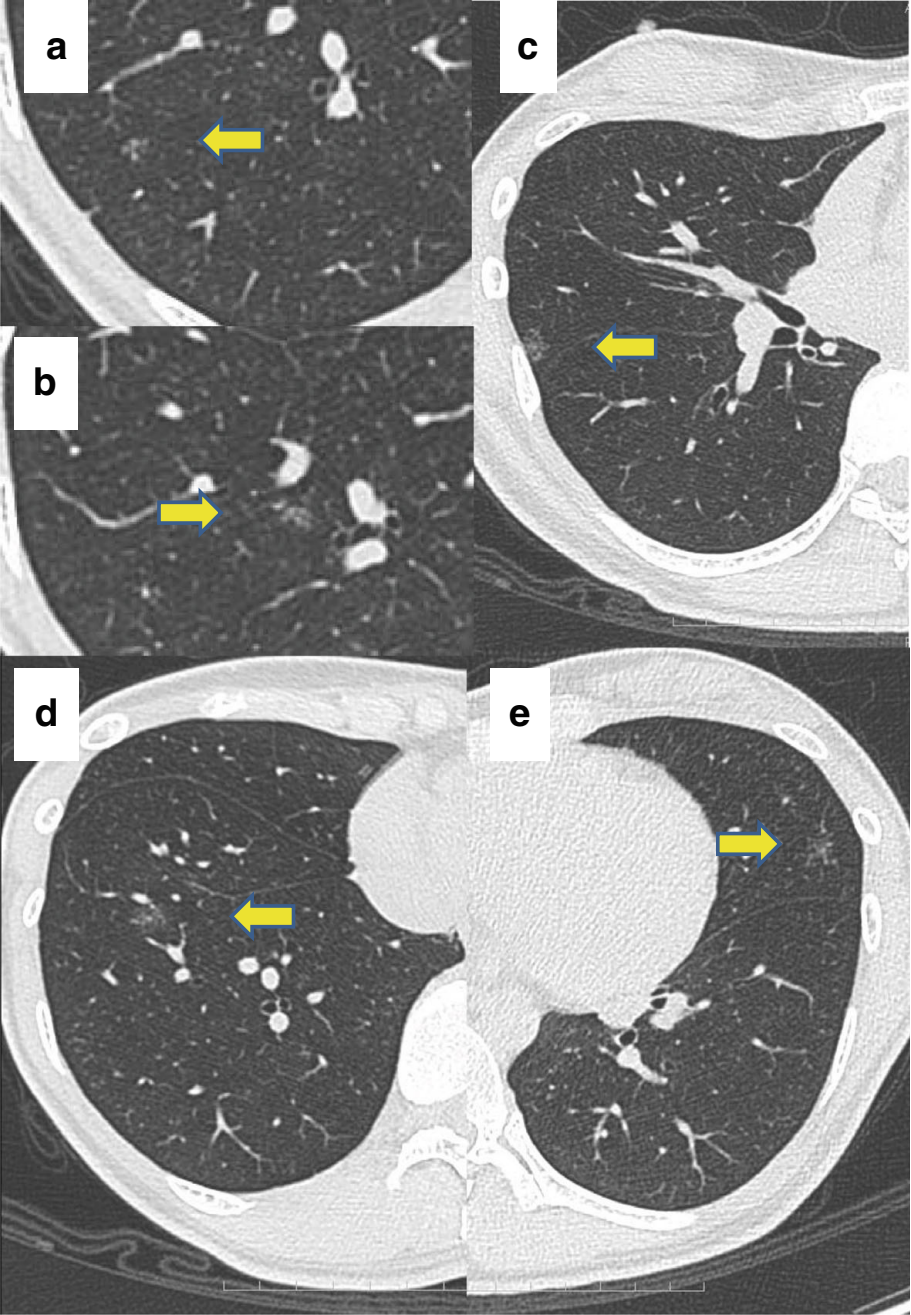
Chest computed tomography in 2015 also revealed multiple shadows with ground glass opacities a, b in right lower lobe, c in right middle lobe, d in segment 8 of right lower lobe, and e in left upper lobe
